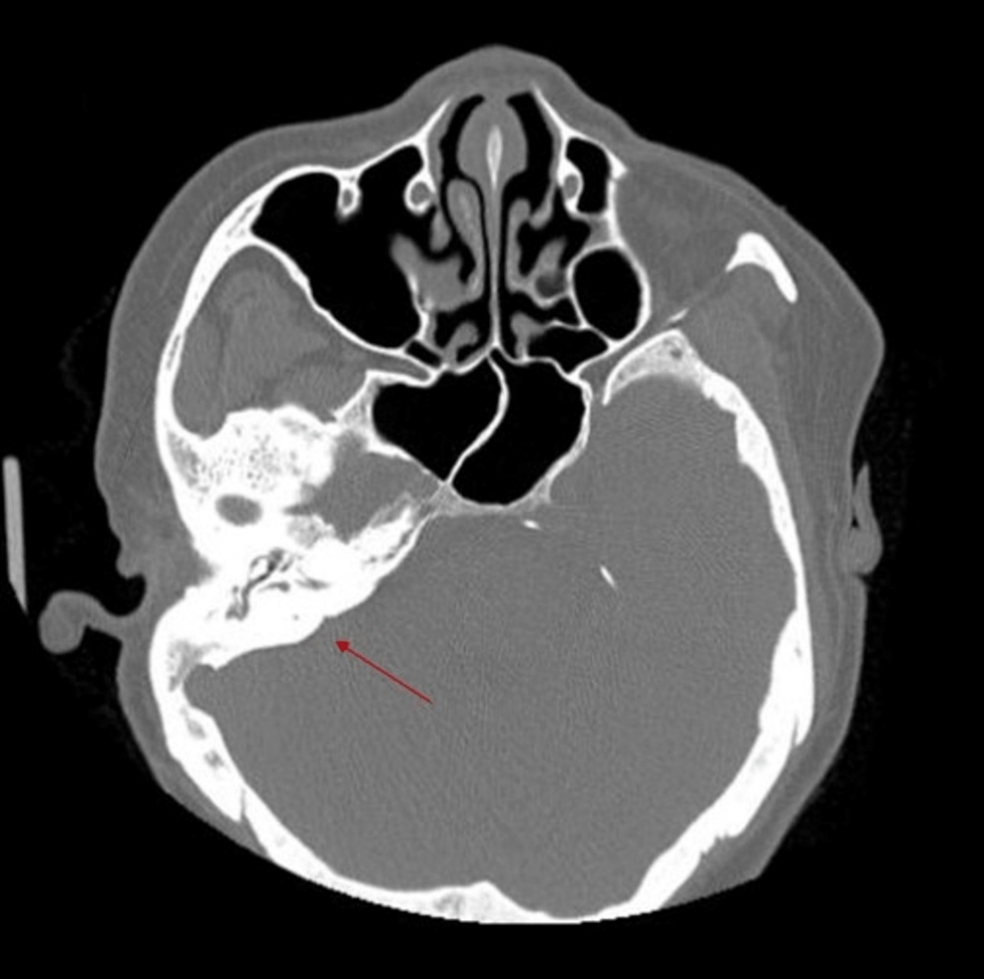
CT paranasal sinus showing right otomastoiditis.
